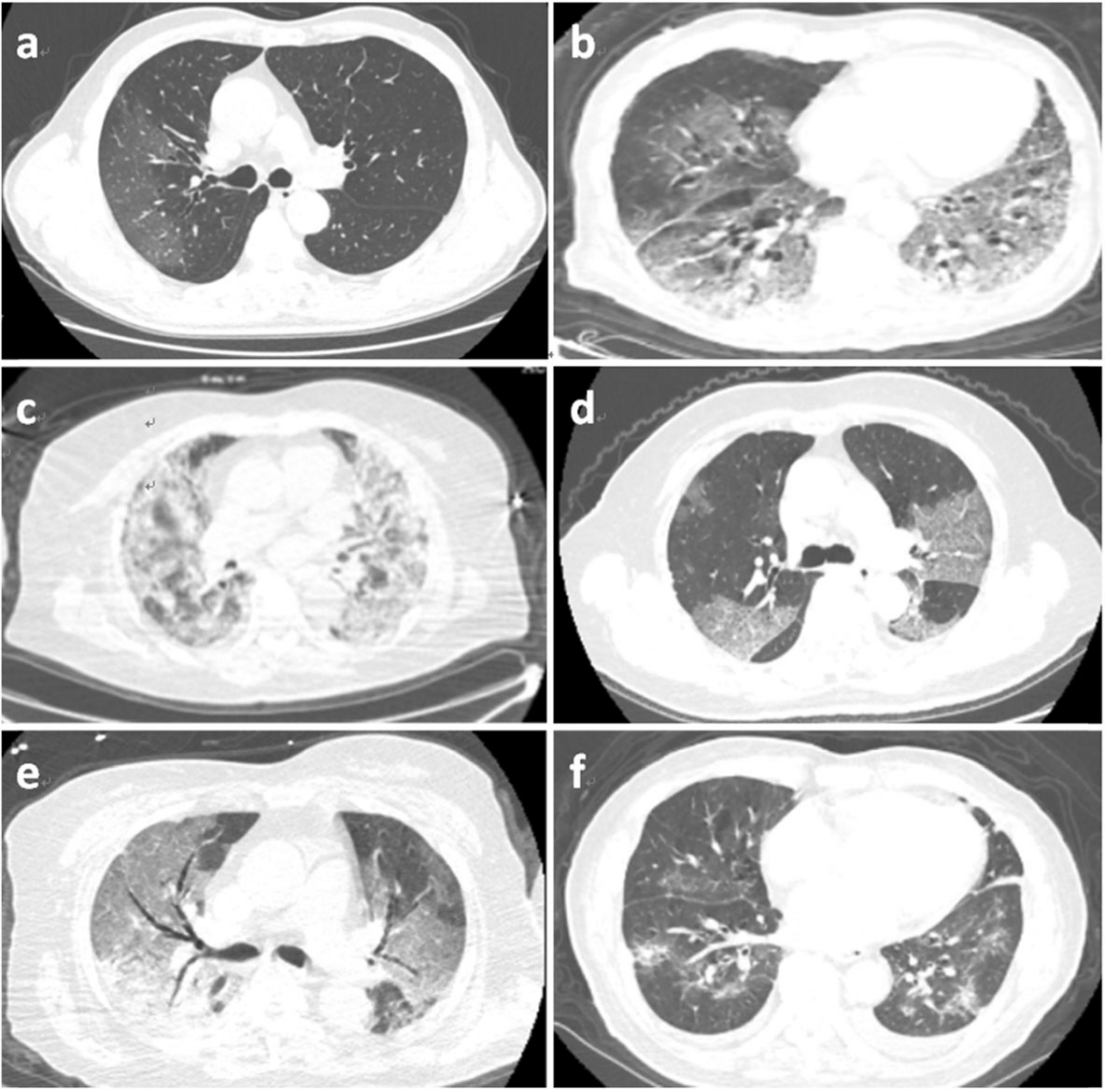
Lung imaging features of axial chest CT scans. a Ground glass opacity(GGO) on the right lung in a patient between 60 and 69 years of age; b Multiple GGO and bronchial dilatation of both lungs in a patient between 60 and 69 years of age; c Multiple GGO and consolidation in a patient between 60 and 69 years of age; d Multiple GGO of both lungs and local consolidation in a patient between 70 and 79 years of age; e Multiple GGO and air bronchogram in a patient between 60 and 69 years of age; f Multiple GGO and pleural effusion in a patient between 80 and 89 years of age
